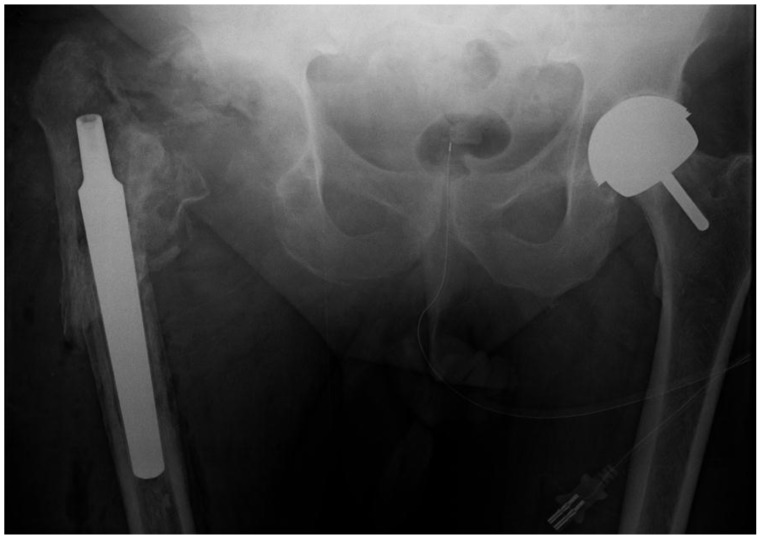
Pelvic x-ray showing the retained femoral stem prior to the second revision.
